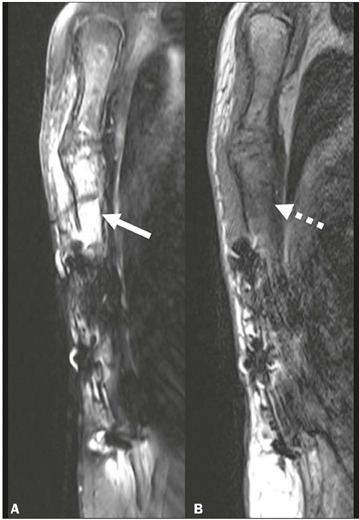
Osteomyelitis depicted by magnetic resonance imaging. A:
